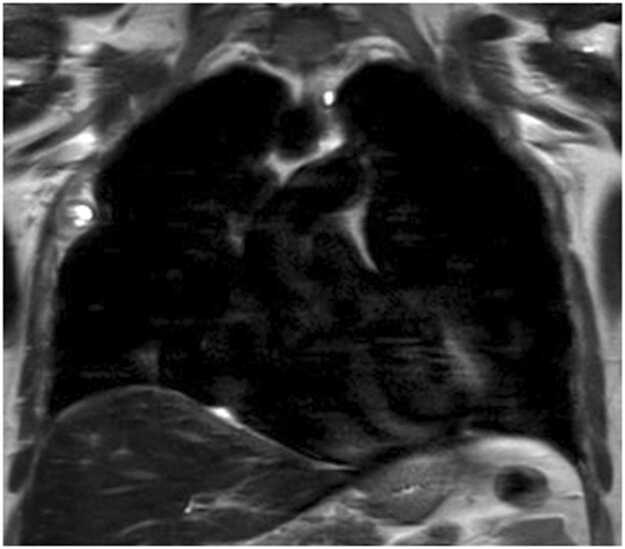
MRI showing primary pleural hydatid cyst: a rare presentation of echinococcosis.
